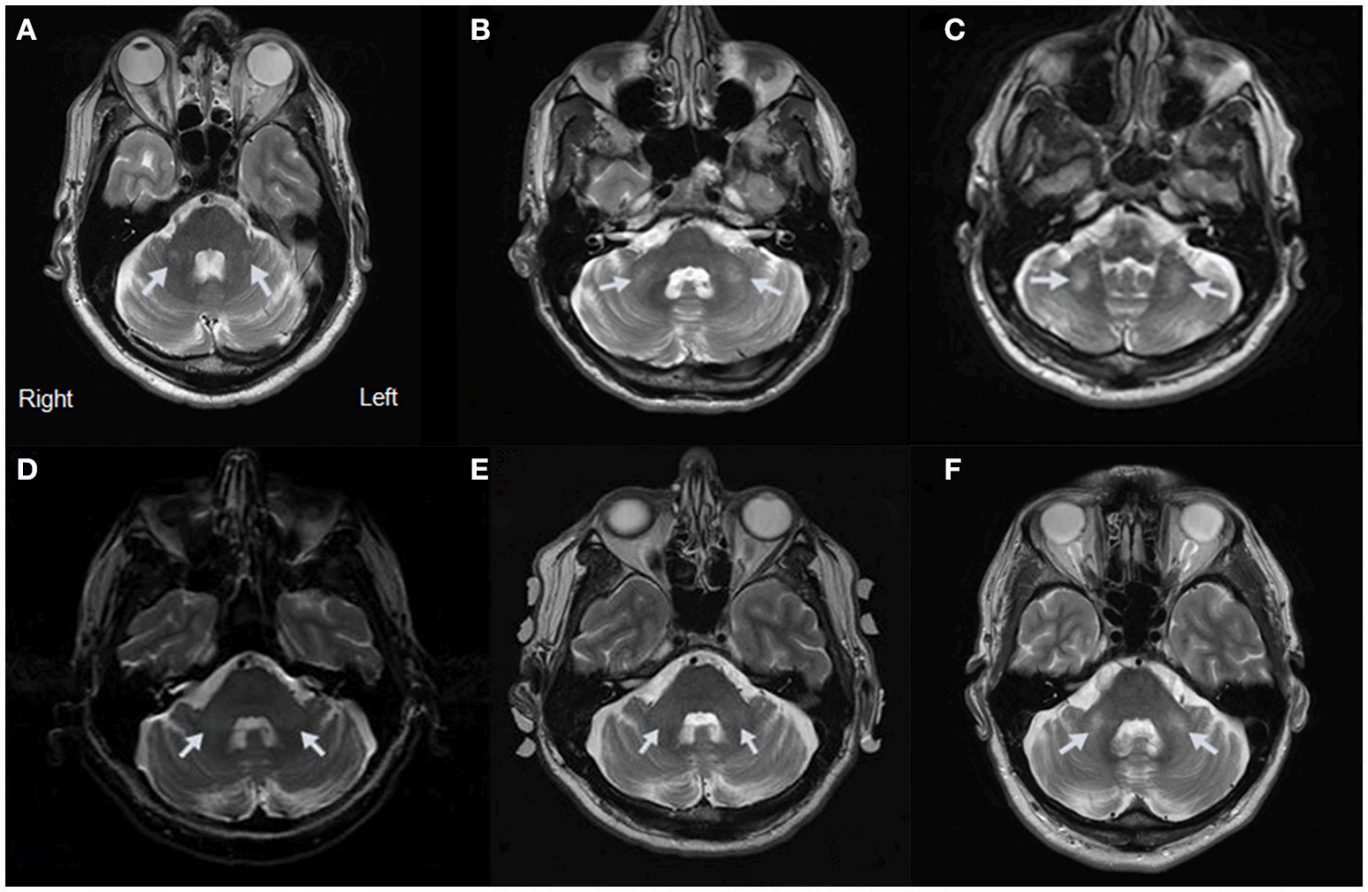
MRI Evidence of MCP Sign for Cases 1–5. (A) Axial view of Case 1 showing a hyperintensity of the MCP. (B) Axial view of Case 2 showing a hyperintensity of the MCP. (C) Axial view of Case 3 showing a hyperintensity of the MCP. (D) Axial view of Case 4 showing a hyperintensity of the MCP at age 60. (E) Axial view of Case 4 showing a hyperintensity of the MCP at age 64. (F) Axial view of Case 5 showing a hyperintensity of the MCP.
